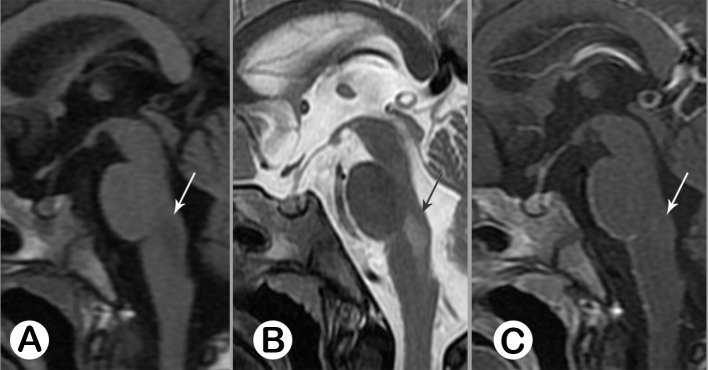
Acute stage typeⅠ.Female, 3 years old, on the 9th day of the disease. Sagittal MR images revealed a longitudinal lesion at the posterior junction region of the pons and medulla oblongata, with prolonged T1 (A, white arrow) and prolonged T2 (B, black arrow) signal, without enhancement (C, white arrow). See follow-up study in Fig 9.
